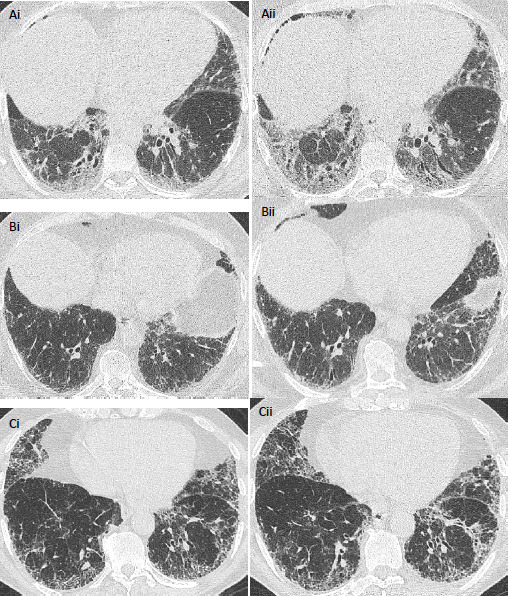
Serial axial CT images in patients with idiopathic pulmonary fibrosis. In a 50-year-old male patient who did not receive antifibrotic medication and who demonstrated a >10% annualised FVC decline, images acquired 6 months apart (Ai, ii) show change in traction bronchiectasis categorised as markedly worsened (score=5) by scoring radiologists. In a 62-year-old male patient who received antifibrotic medication (Bi, ii), images acquired 13 months apart show annualised FVC decline between 5.0% and 9.9%, and change in traction bronchiectasis was categorised as mildly worsened (score=4). In a 77-year-old man who did not receive antifibrotic medication (Ci, ii) and who had CTs acquired 15 months apart, change in traction bronchiectasis severity (Score=3) and annualised FVC decline (−5.0% to 4.9%) were both considered stable. Parenchymal changes visible on the CT may reflect disease maturation rather than disease progression. FVC, forced vital capacity.
